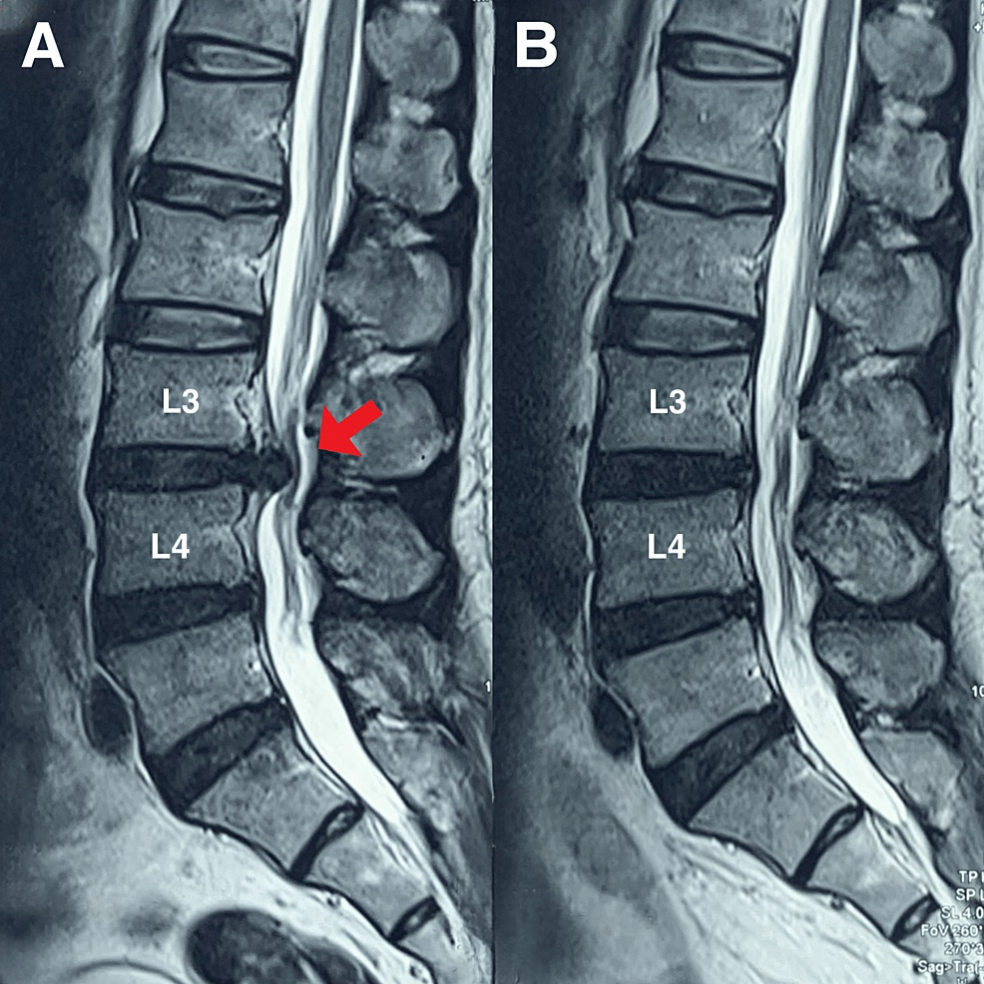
T2 MRI of the lumbar spine in sagittal view (A) The sagittal view revealed a central disc extrusion at L3/L4 measuring 12 × 8 × 6 mm (red arrow), causing severe spinal canal stenosis and compression of the descending L4 nerve roots bilaterally within the central canal. Mild disc bulges were noted at the L4/L5 and L5/S1 levels without significant stenosis. (B) A follow-up MRI of the lumbar spine revealed resolution of the L3/L4 disc extrusion.
